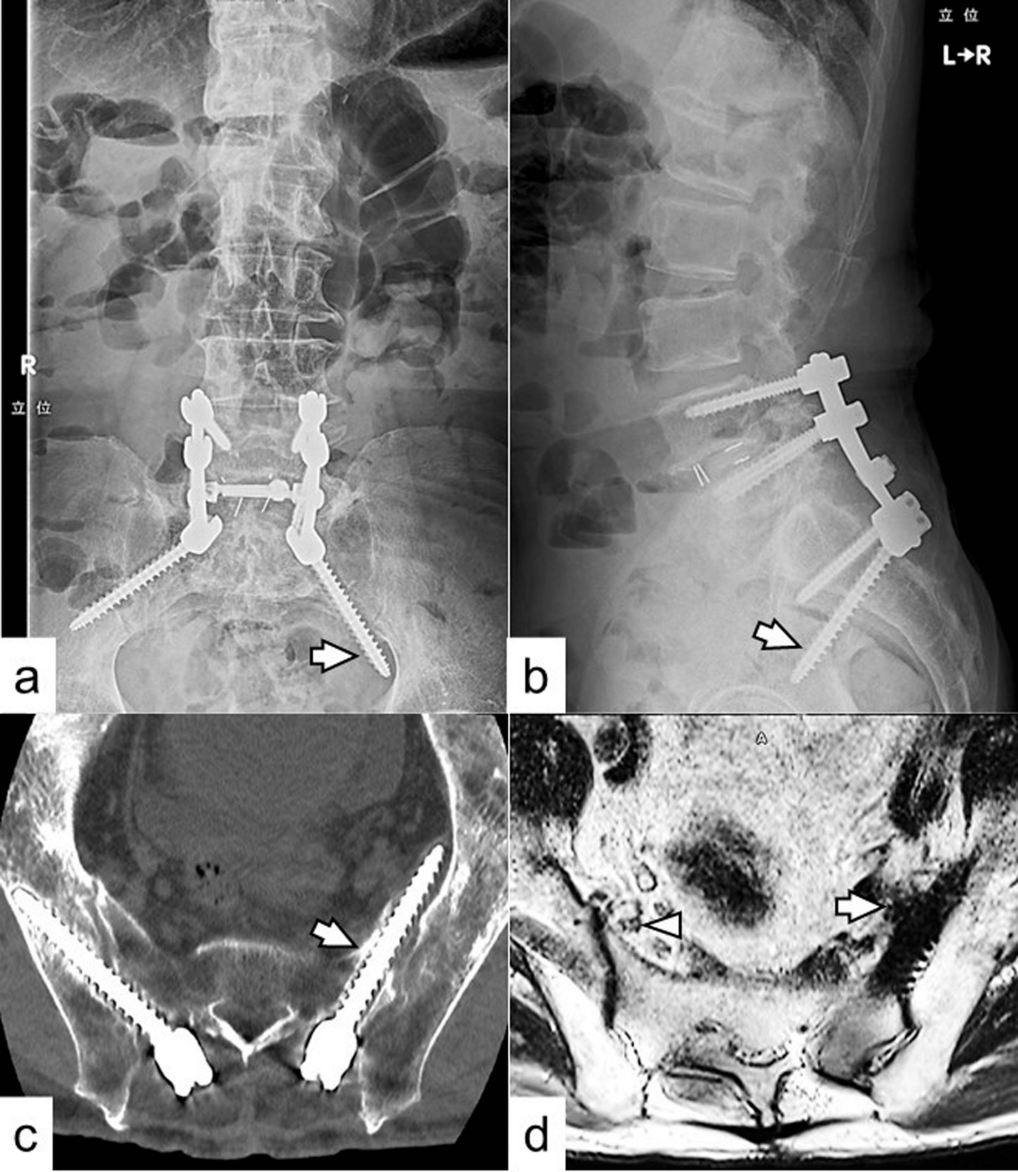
Imaging findings during the patient’s first visit to our institute. Anteroposterior (a) and lateral (b) views of the lumbosacral spinal plain radiography reveal a caudally misplaced S2AI screw on the left side (arrow). (c) CT shows the left S2AI screw severely protruding into the pelvic cavity and breaching the anterior cortex of the sacrum (arrow). (d) T2-weighted MRI reveals that the left L5 spinal nerve is markedly displaced anteriorly by the misplaced S2AI screw (arrow) compared to the right L5 spinal nerve, which is located just medial to the inferior sacroiliac joint (arrowhead). S2AI, S2 alar-iliac; CT, computed tomography; MRI, magnetic resonance imaging
